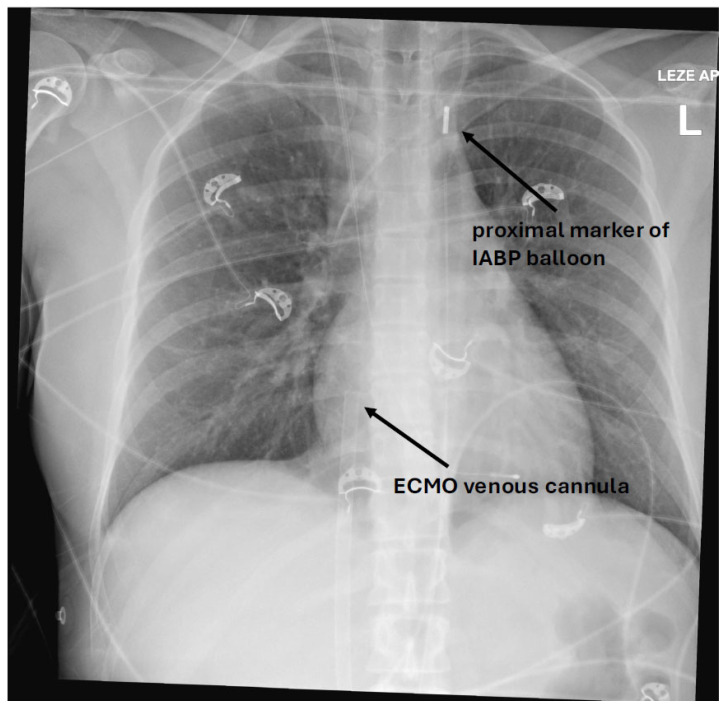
X-ray of a patient showing a proximal IABP balloon marker in aortic knob and an ECMO venous cannula with the tip in the right atrium.
